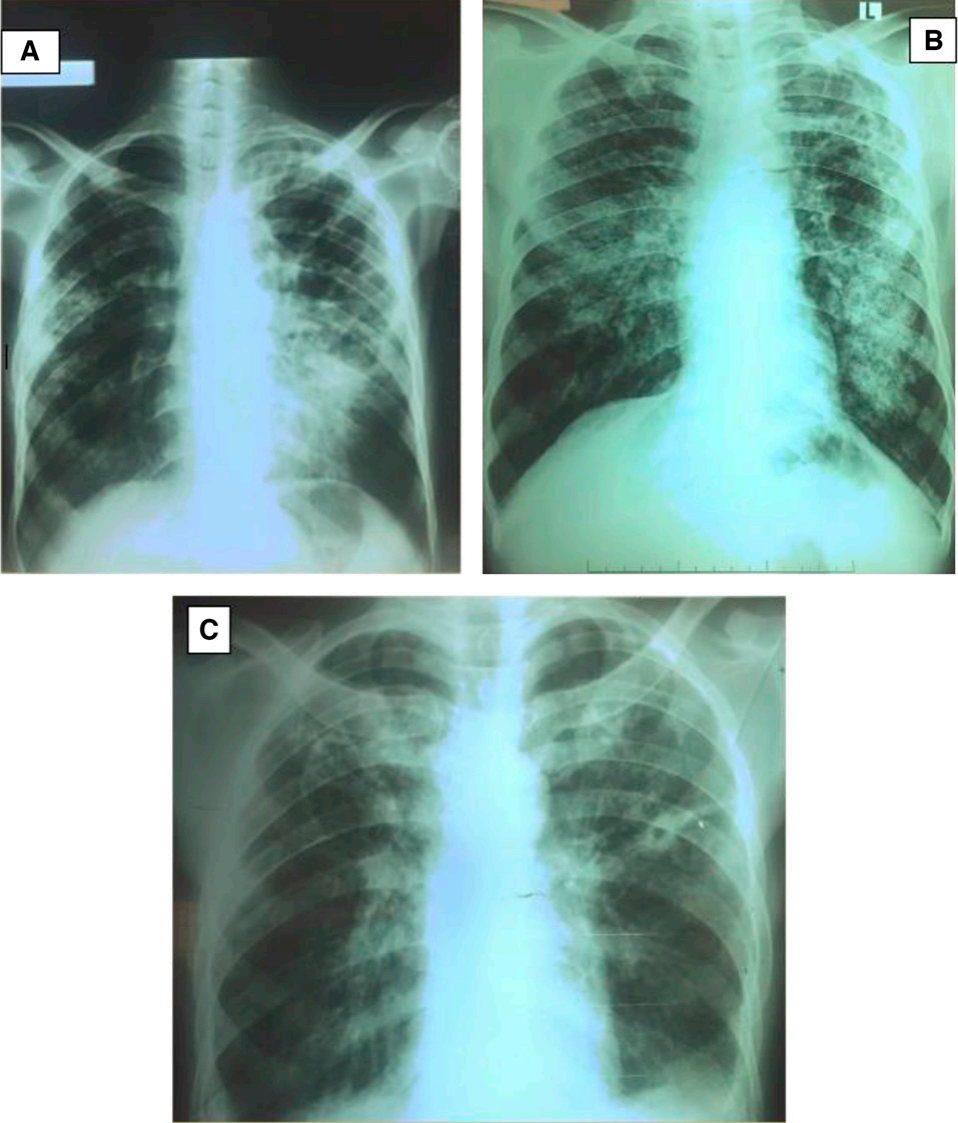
Chest radiographs of the three patients who had severe COVID-19 and tuberculosis. (A) Left lung consolidation and fibrosis in a 18 years old male. (B) Bilateral lung infiltrates in a 40-year-old male. (C) Hilar lymphadenopathy, right apical infiltrates and left lung cavitation in a 28-year-old male.
